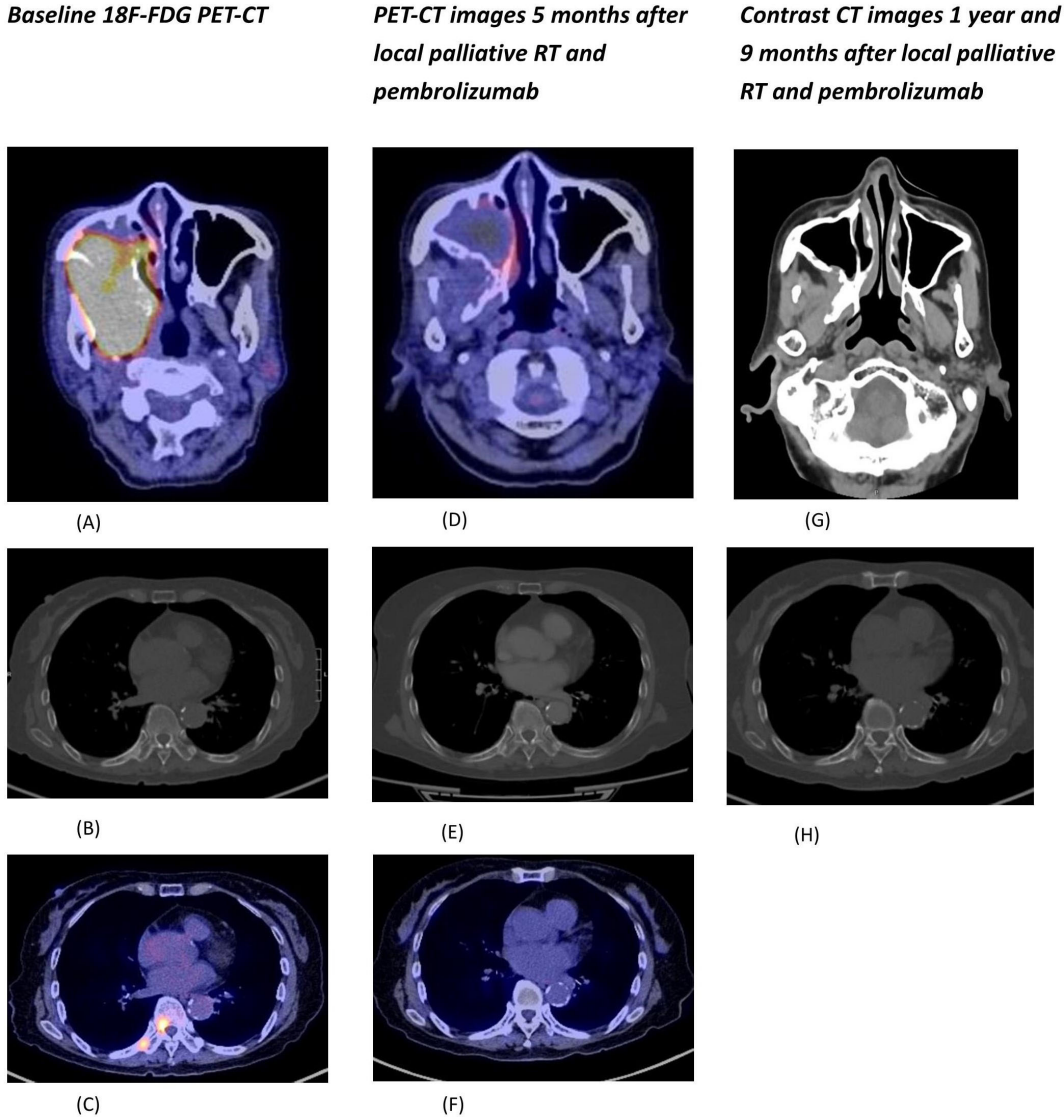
(A) Fusion PET-CT of the hypermetabolic primary tumor involving the right maxillary sinus and right parapharyngeal region. (B) CT (bone window) showing sclerotic bone metastases at right T9 and right 9th rib. (C) Fusion PET-CT of hypermetabolic bone metastases at right T9 and right 9th rib. (D) Fusion PET-CT of the primary tumor showing significant shrinkage and metabolic response. (E) CT (bone window) of lesions at right T9 and right 9th rib with radiological improvement. (F) Fusion PET-CT of lesions at right T9 and right 9th rib with metabolic response. (G) Contrast CT (soft tissue window) showing sustained tumor response with only residual mucosal thickening at the posterior wall of right maxillary sinus. (H) CT (bone window) of lesions at right T9 and right 9th rib with further radiological improvement.
